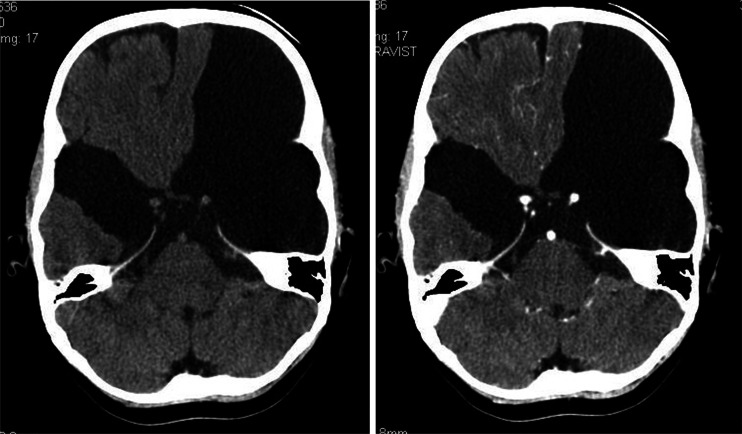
Arachnoid cysts of the lateral sulcus, the larger one being situated on the left side, a minimal mass effect. Nonenhanced CT (Fig. 1) contrast-enhanced CT (Fig. 2)
